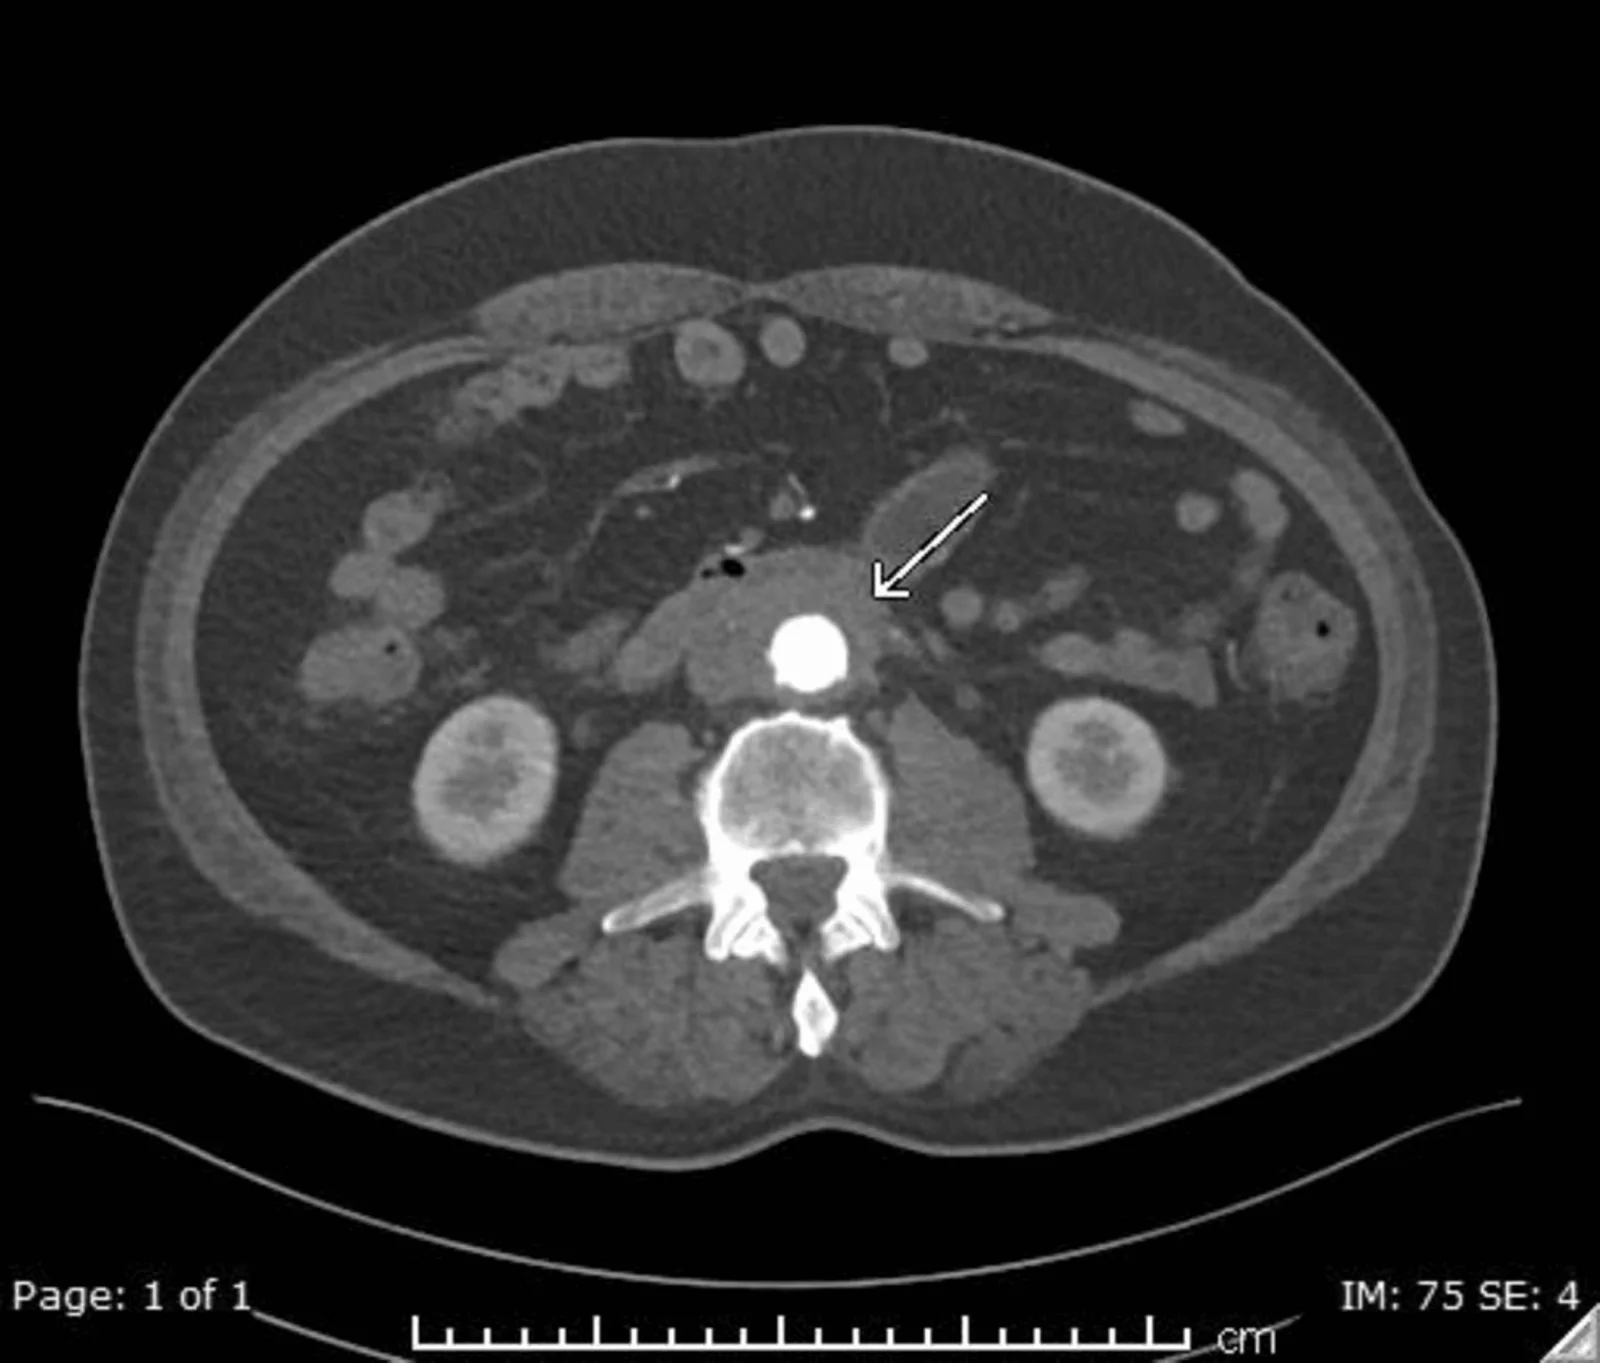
CT angiography of the abdomen (coronal plane) showing periaortic inflammation (arrow)
